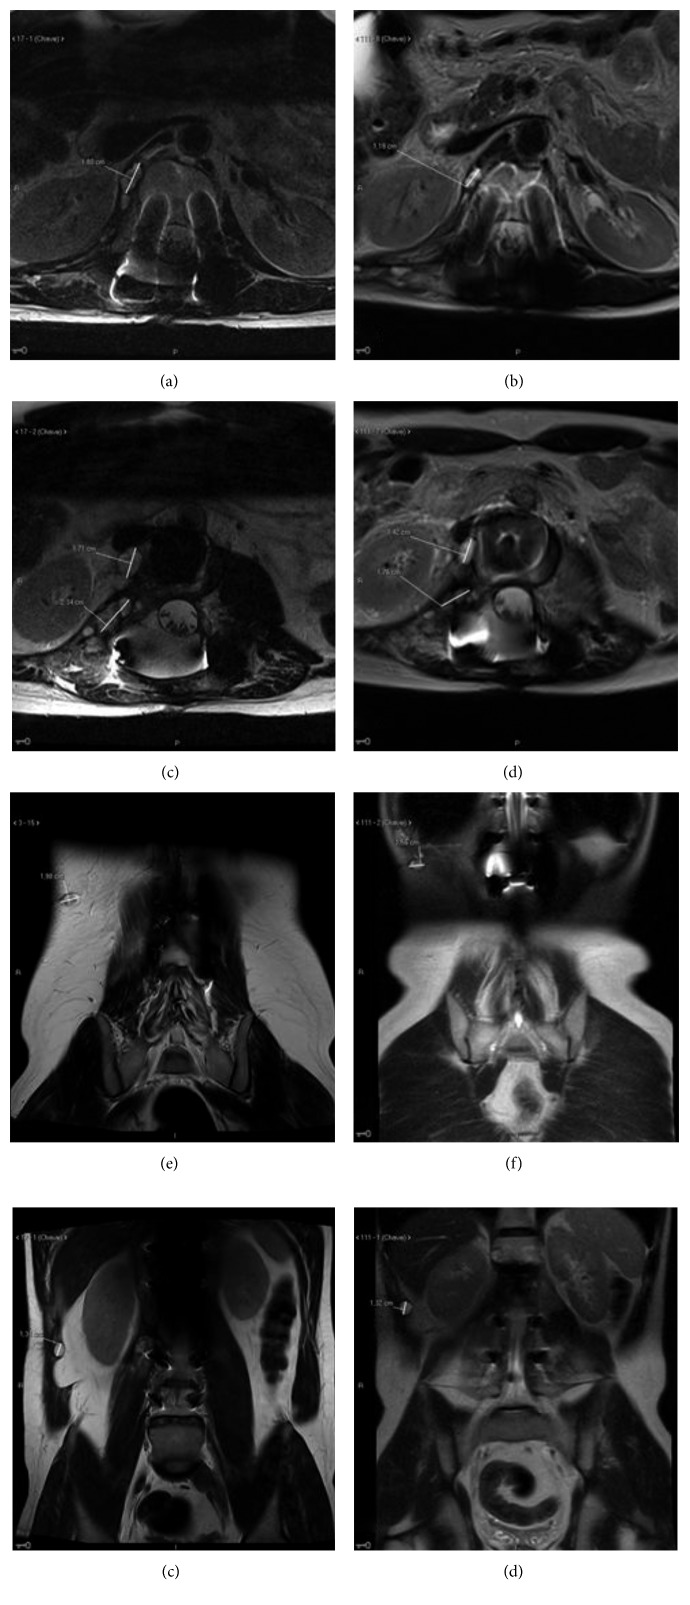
Baseline and posttreatment imaging showing multifocal recurrence of spinal chordoma and tumor reduction following treatment with pazopanib. In the left column (a, c, e, and g): baseline lumbar spine MRI. In the right column (b, d, f, and h): follow-up abdominal MRI.
